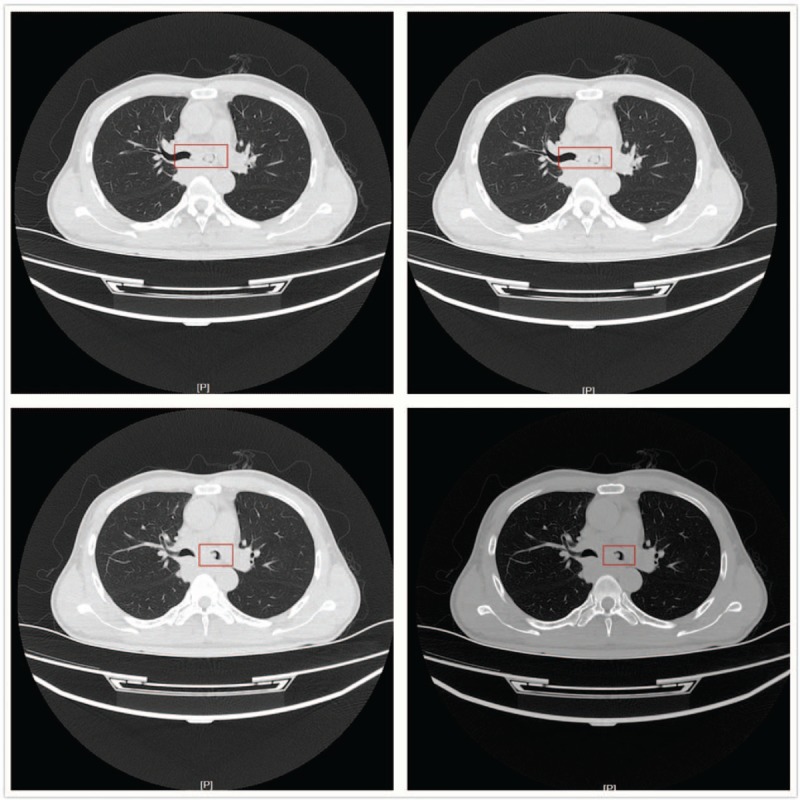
Chest CT before operation. CT = computed tomography.
